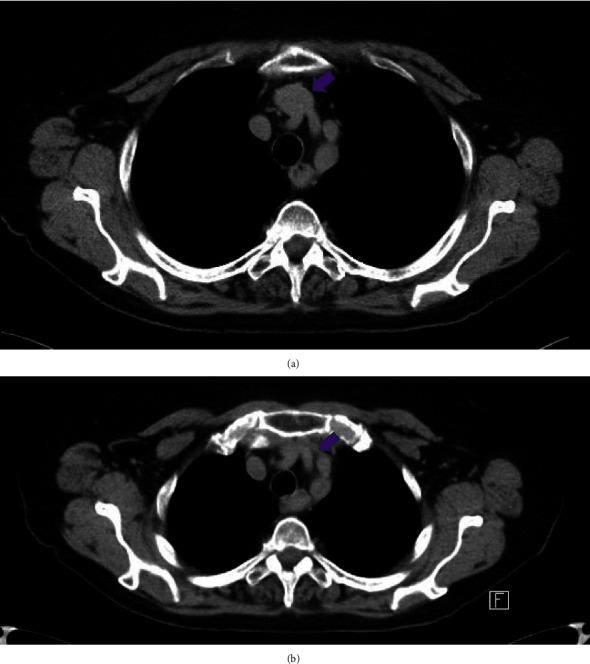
(a) Axial noncontrast CT obtained in full inspiration demonstrates patent left brachiocephalic vein (purple arrow). AP chest diameter: 8 cm. (b) Axial noncontrast CT obtained in full expiration demonstrates stenosis of the left brachiocephalic vein (purple arrow) as a result of compression between the left common carotid artery/left brachiocephalic artery and the manubrium. AP chest diameter: 5.6 cm.
